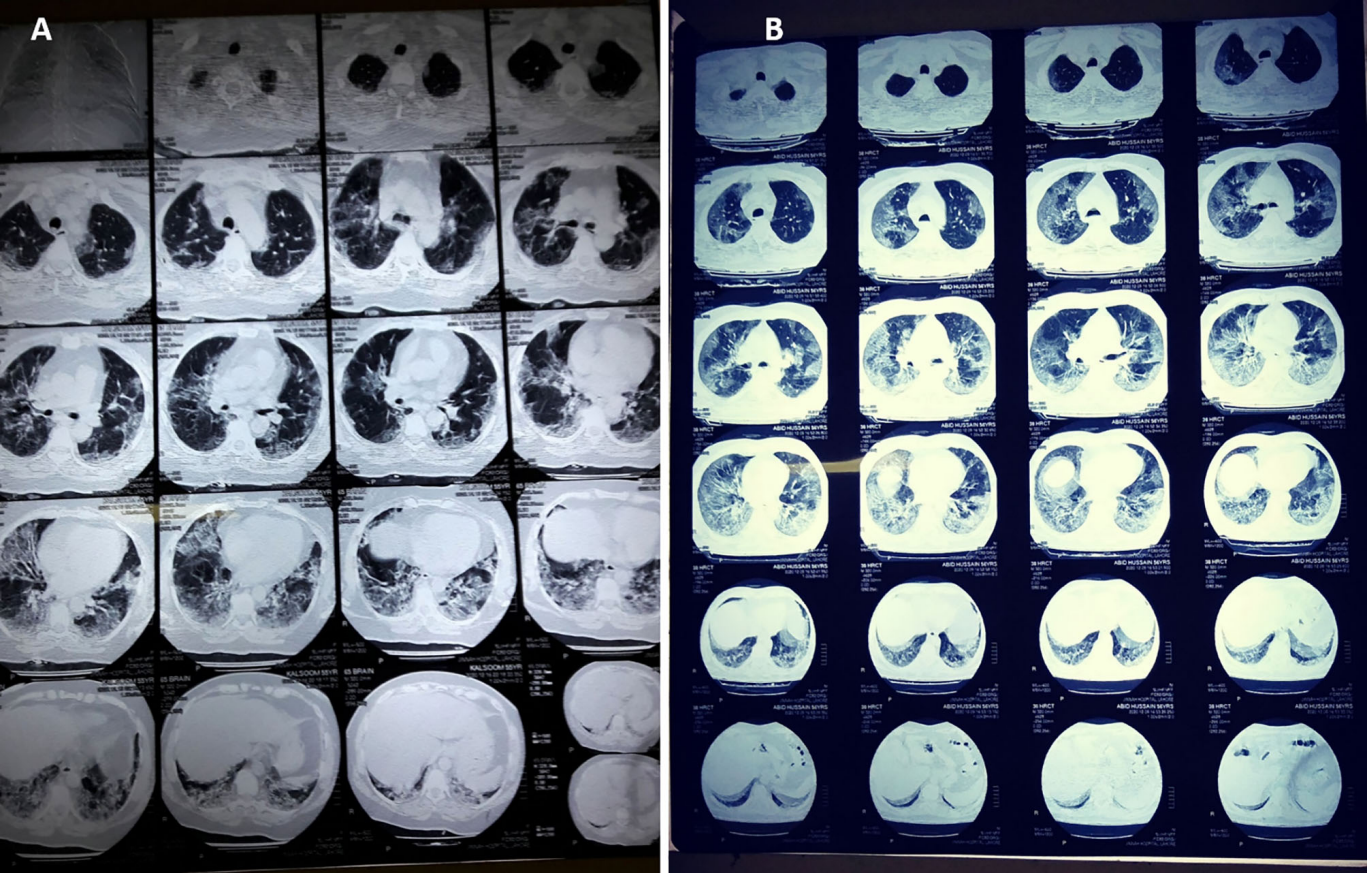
CT scan digital images of (A) mild-to-moderate and (B) severe SARS-CoV-2-infected patients. The percentage of lung involvement can be spotted significantly in both types of patient.
